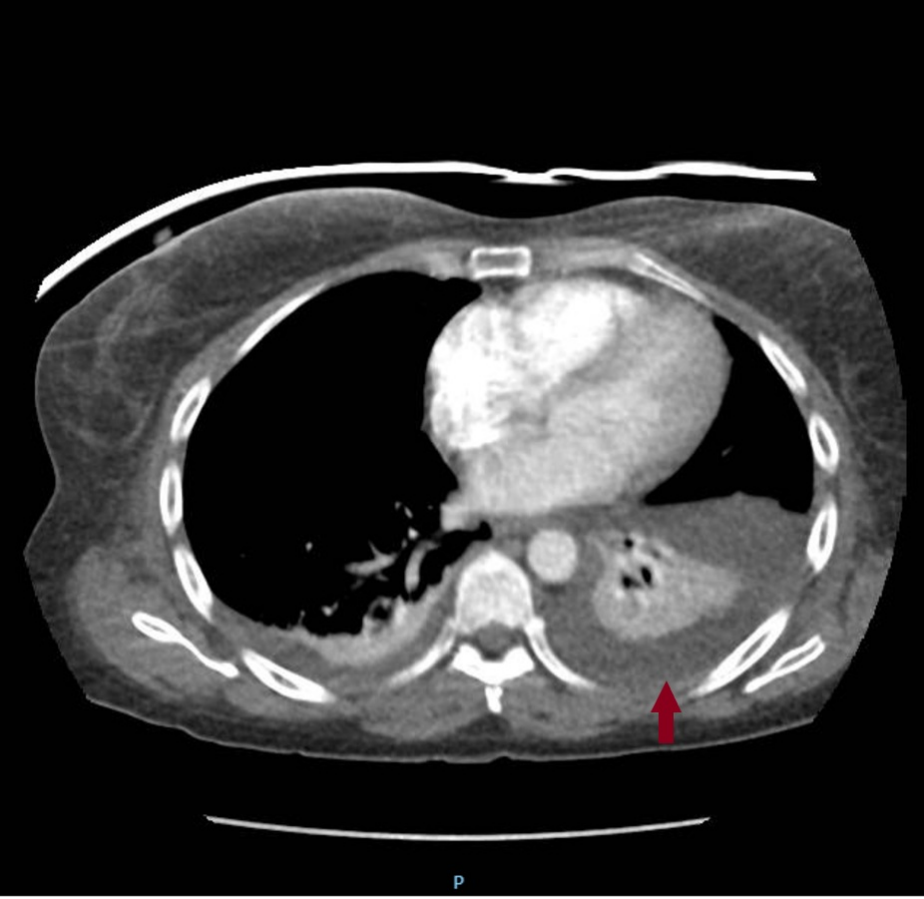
CT scan of chest showing left-sided pleural effusion. CT: Computed tomography
